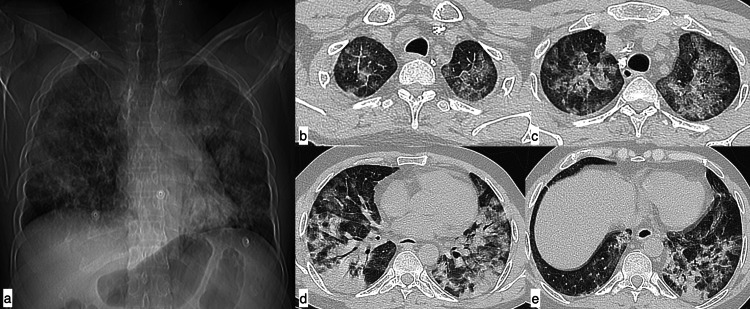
Infective aetiology - polymicrobial infection A 40-year-old male patient with SLE presented with fever, shortness of breath, chest pain, and cough of 11-day duration. The topogram (a) showed patchy consolidations in bilateral lung fields. Axial HRCT chest images in the lung window (b-e) showed interlobular septal thickening with ground-glass opacities with patchy areas of consolidation in bilateral lung fields. Diagnosis of infective aetiology was considered, which was later confirmed as polymicrobial infection (Pneumocystis carinii, bacterial). SLE: systemic lupus erythematosus; HRCT: high-resolution CT
